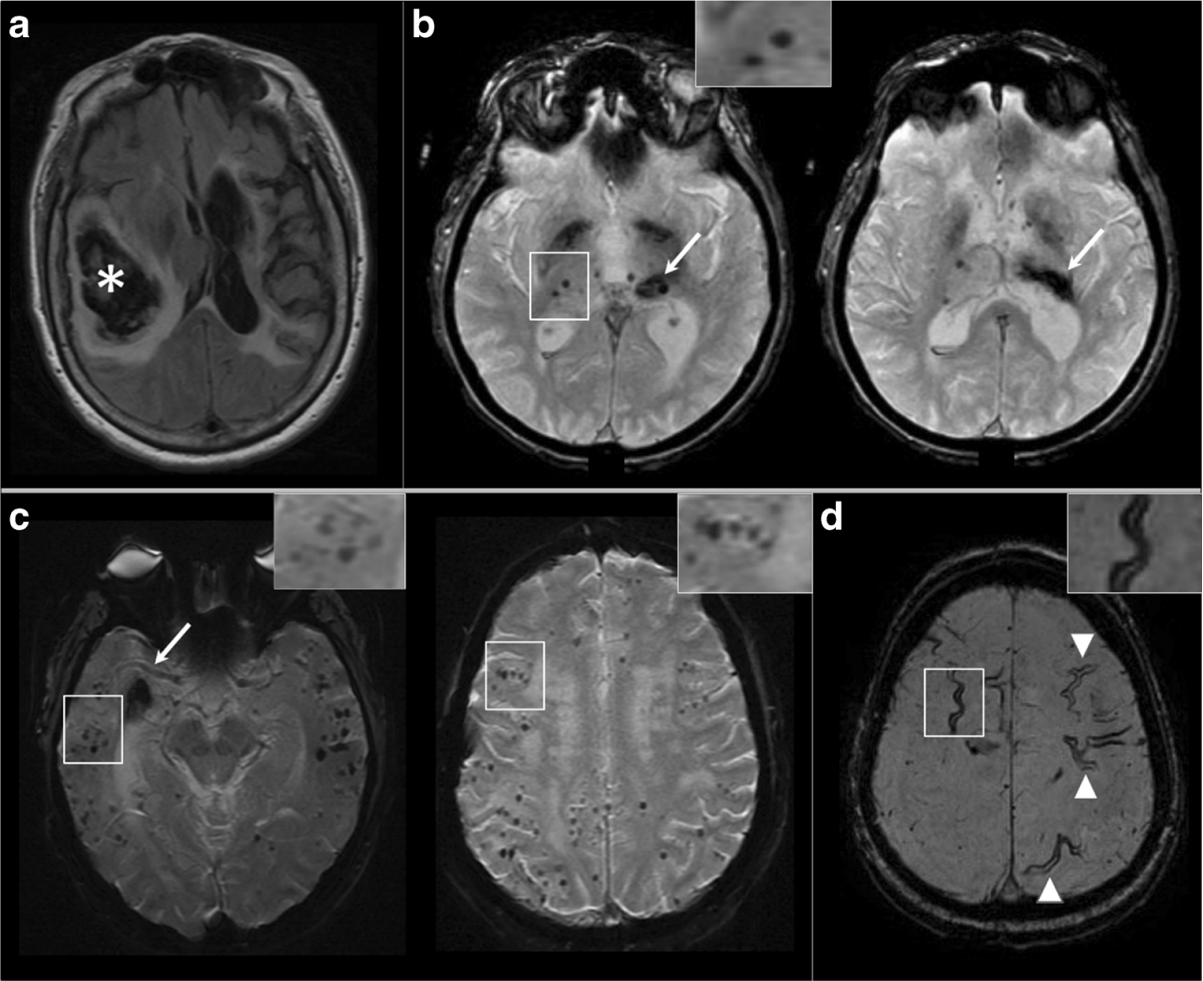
Hemorrhage-prone small vessel disease markers. a Axial FLAIR sequence; large right parieto-temporal hematoma with extensive edema causing midline shift (star) and also extensive periventricular white matter hyperintensities. b Axial T2* sequence showing a patient with a left thalamic hematoma (arrows) and bilateral deep cerebral microbleeds (inset). c Axial T2* sequences showing many strictly lobar microbleeds (inset) in a patient with a right temporal hematoma. According to the Boston criteria, this patient fulfills criteria for cerebral amyloid angiopathy. d Extensive cortical superficial siderosis (arrowheads and inset) visible in T2* sequence
